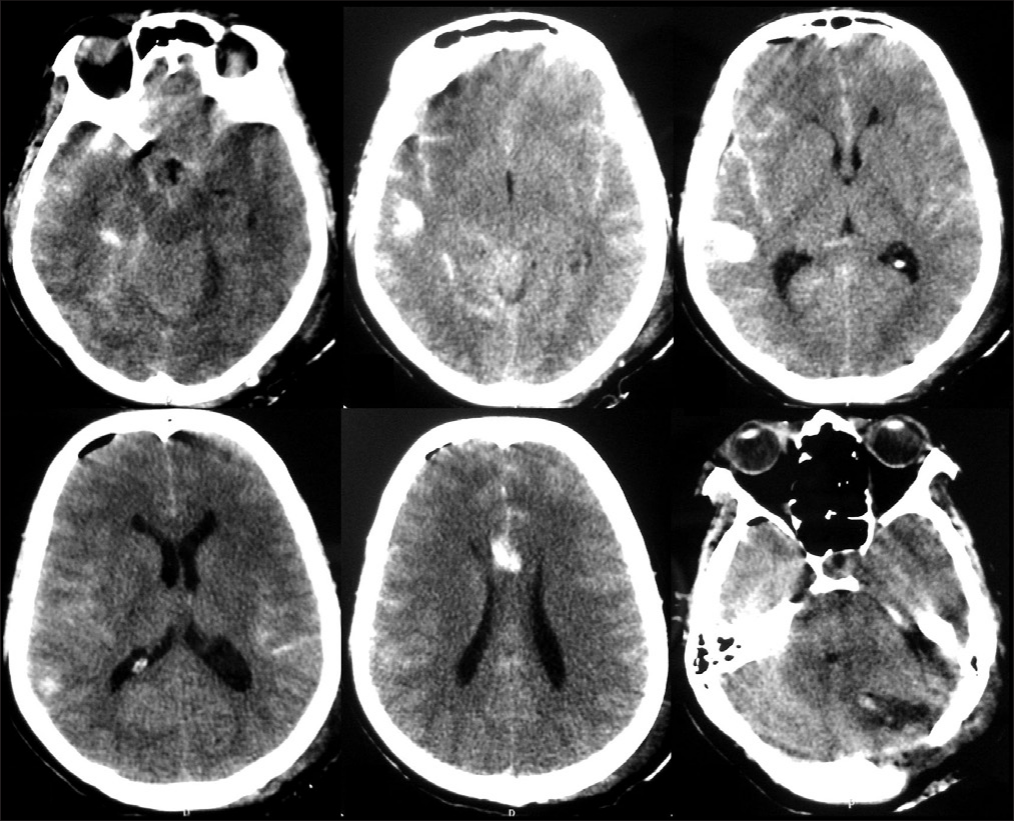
Postoperative CT scan demonstrates extensive subarachnoid hemorrhage with diffuse cerebral edema
